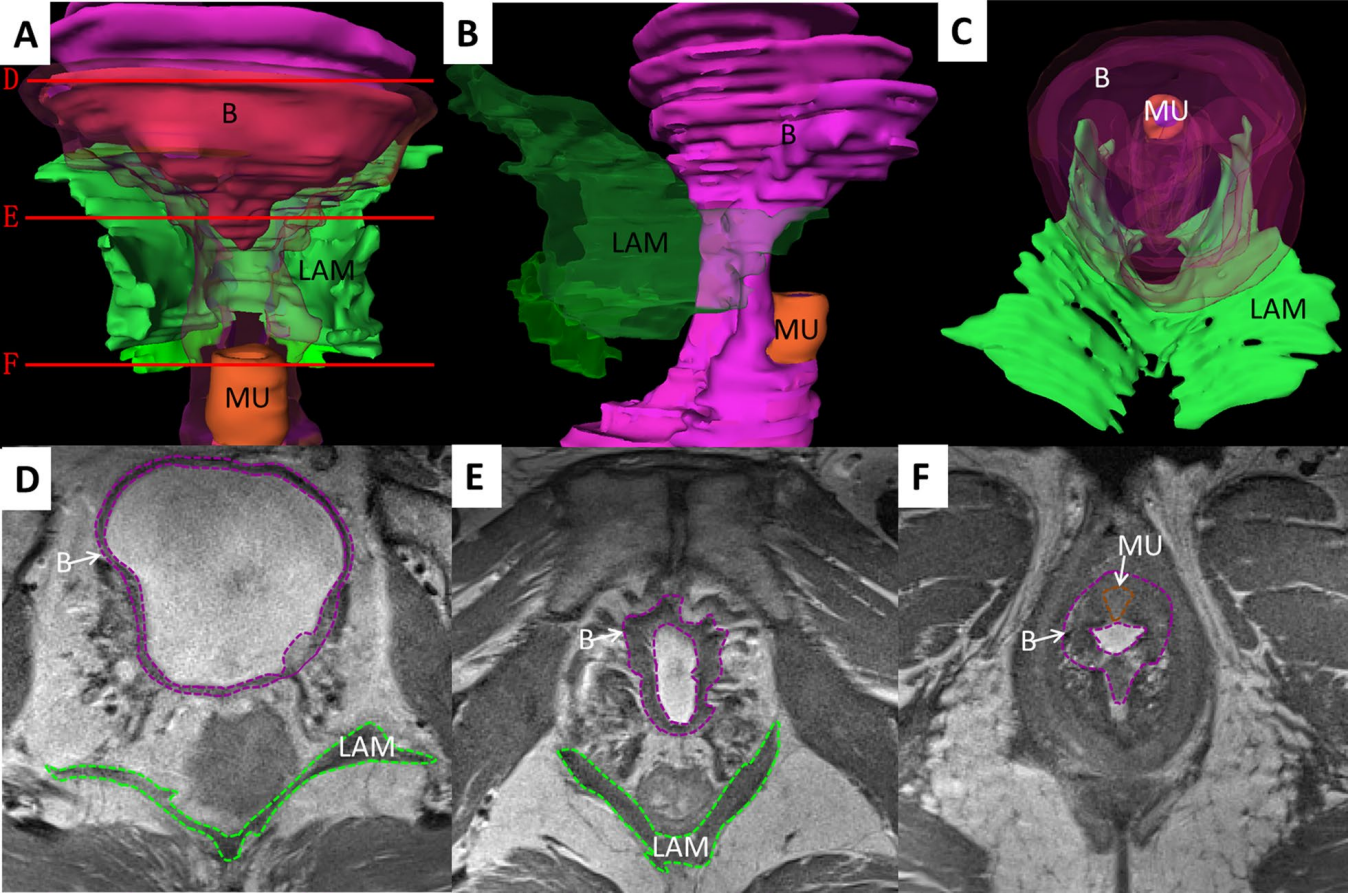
3D models and transverse MRI images of bladder detrusor and urinary continence components of POP patients. A Anterior view; B Left view; C Superior view; D Bladder section; E Superior urethra section; F Inferior urethra section. LAM, levator ani muscle; B, bladder detrusor; MU, main part of urethral sphincter
